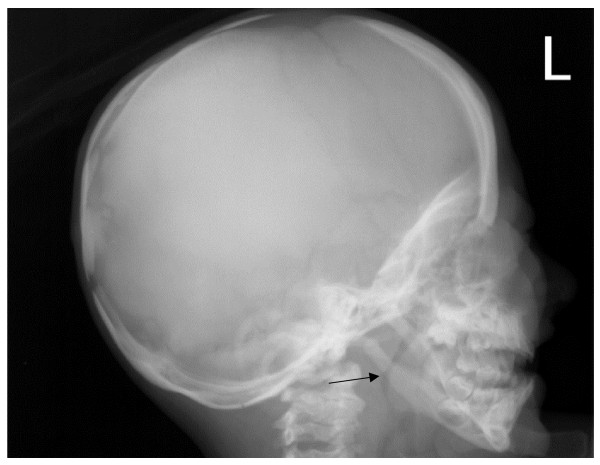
Pycnodysostosis: Lateral skull radiograph, age 3 years. Note loss of the mandibular angle (arrow) and increased thickness of vault.
